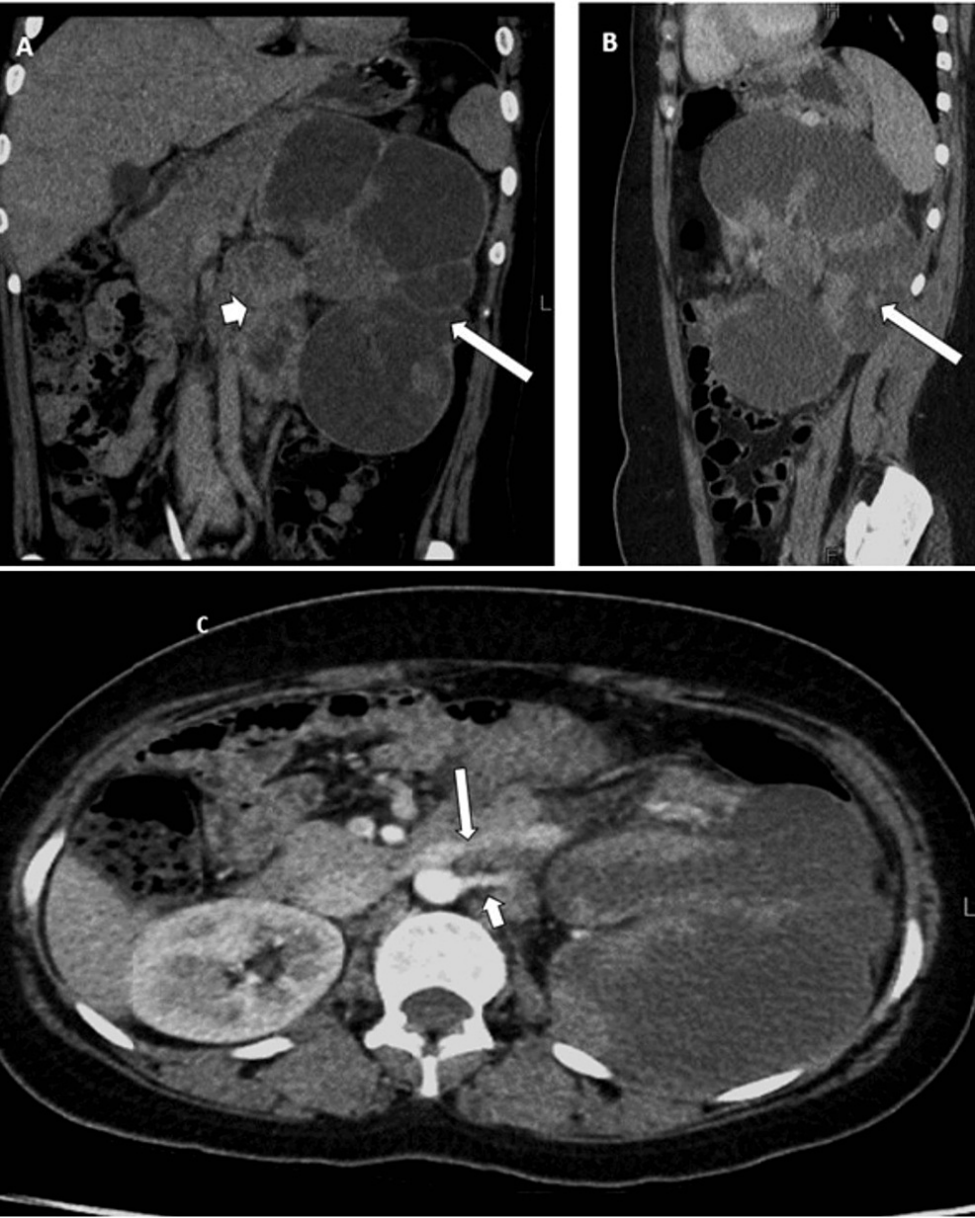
An 18-year-old female presented with left flank pain and weight loss for one month (A, B) Post-contrast CT scans revealed a large reniform multiloculated cystic lesion with solid-enhancing components, completely replacing the entire left kidney (long arrow). Additionally, conglomerate partly necrotic paraaortic lymph nodes were observed (short arrow). (C) An axial image showed the left renal artery (short arrow) and vein (long arrow) encased by the lymph nodal mass.
